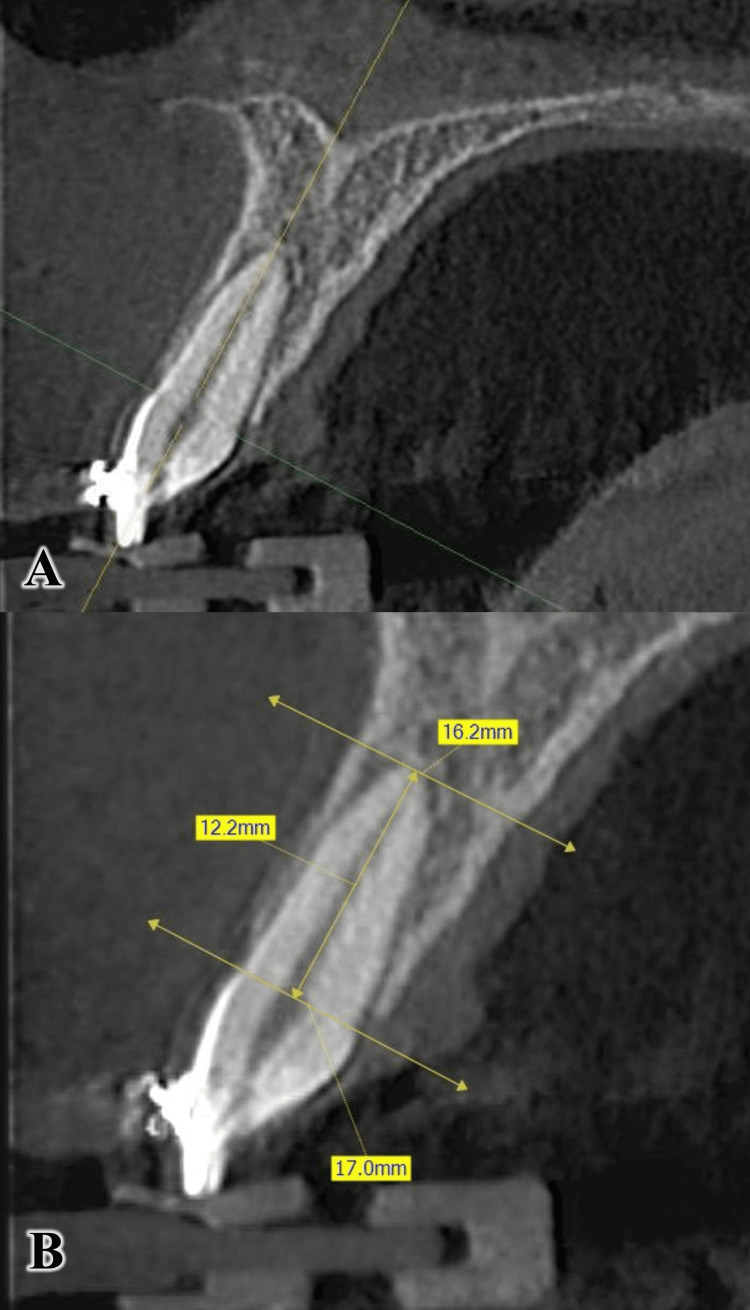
Calculating the root length on CBCT images in the sagittal view. A: Orientation of the two axes (i.e., the frontal and the axial) on the sagittal view. B: Measuring the distance between the two parallel yellow lines to determine the root length CBCT: cone-beam computed tomography.
